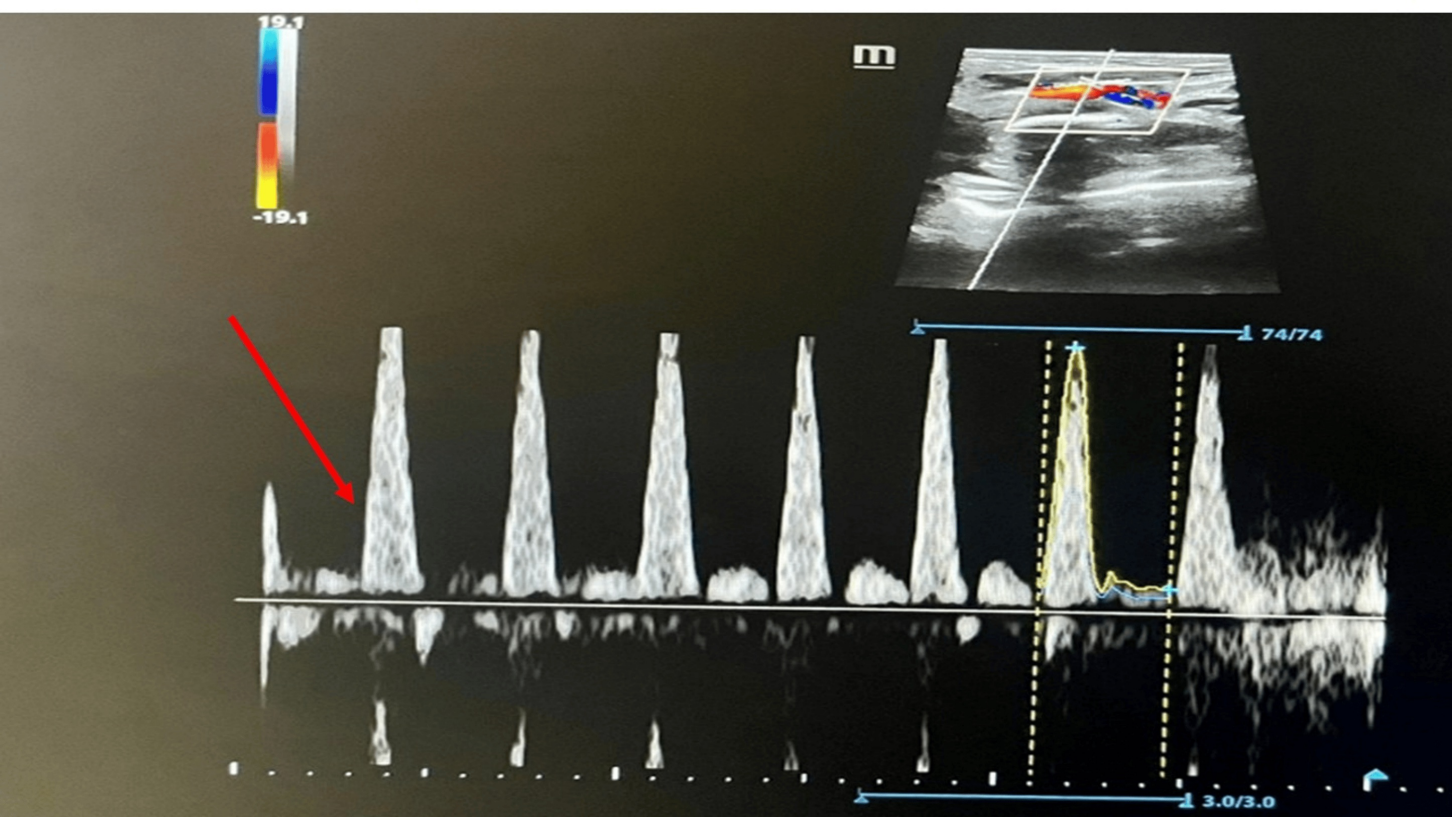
Right femoral artery Doppler showing normal triphasic waveforms (red arrow)
